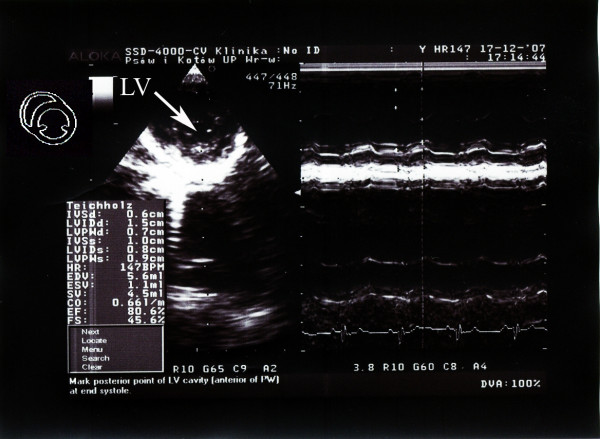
Echocardiographic image. Example of an echocardiographic examination in (Parasternal projection in the short axis) a European brown hare anaesthetized by xylazine and ketamine. Left ventricle: LV.
